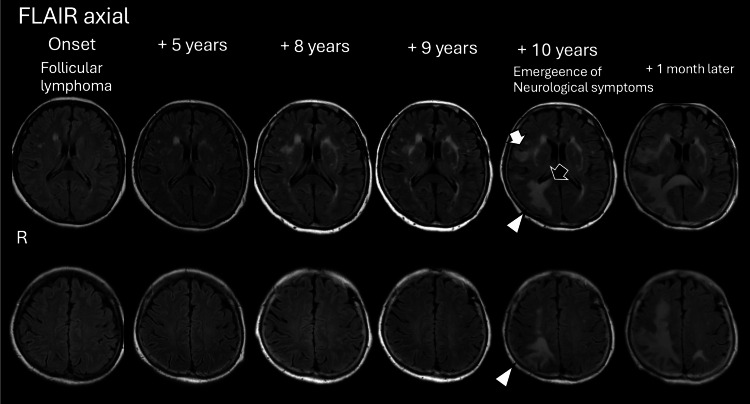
Longitudinal changes of white matter lesions including progressive multifocal leukoencephalopathy (PML) on the brain MRI. Axial FLAIR images are shown from the first onset of follicular lymphoma to PML. When screening brain imaging at FL onset, the patient presented with ischemic patches in the right subcortical white matter and periventricular regions. Due to an incidentally found aneurysm in the left internal carotid artery and clipping at five years after FL onset, a brain MRI was performed annually. At five, eight, and nine years after the onset of FL, ischemic lesions increased. At 10 years from onset, neurological symptoms emerged, and the new lesions of PML seemed to overlap with right ischemic lesions, exhibiting homogeneous quality with swelling (white arrow) and involving the right optic radiation, parietal cortices (arrowhead), and corpus callosum (black arrow). Only a month after the onset of neurological symptoms, the subcortical lesions rapidly widened, and the left side lesion emerged. PML: progressive multifocal leukoencephalopathy; FLAIR: fluid-attenuated inversion recovery; FL: follicular lymphoma; MRI: magnetic resonance imaging.
